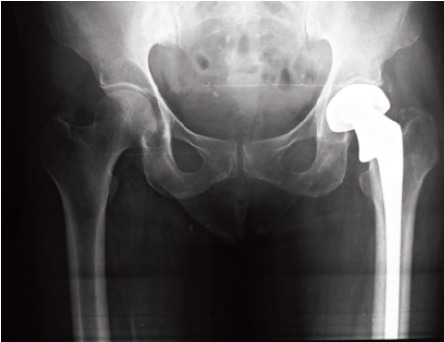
Post operative radiograph of bipolar prosthesis.
